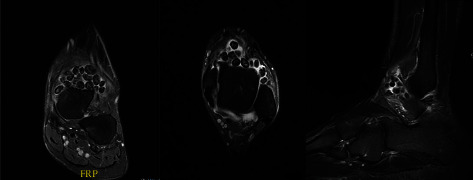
MRI demonstrating coronal, axial, and sagittal views of the ankle.
